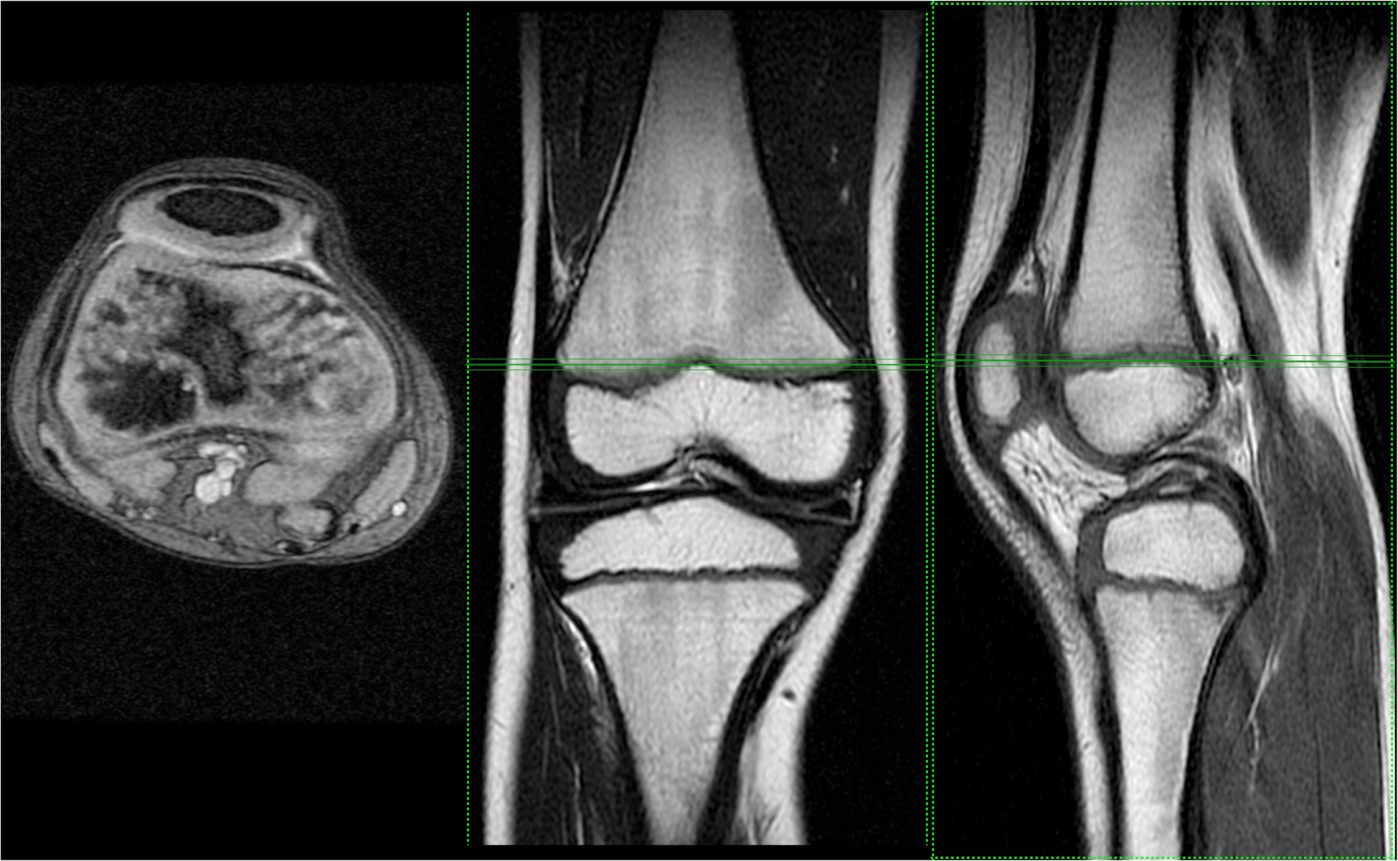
Knee MRI at the femoral growth plate level
